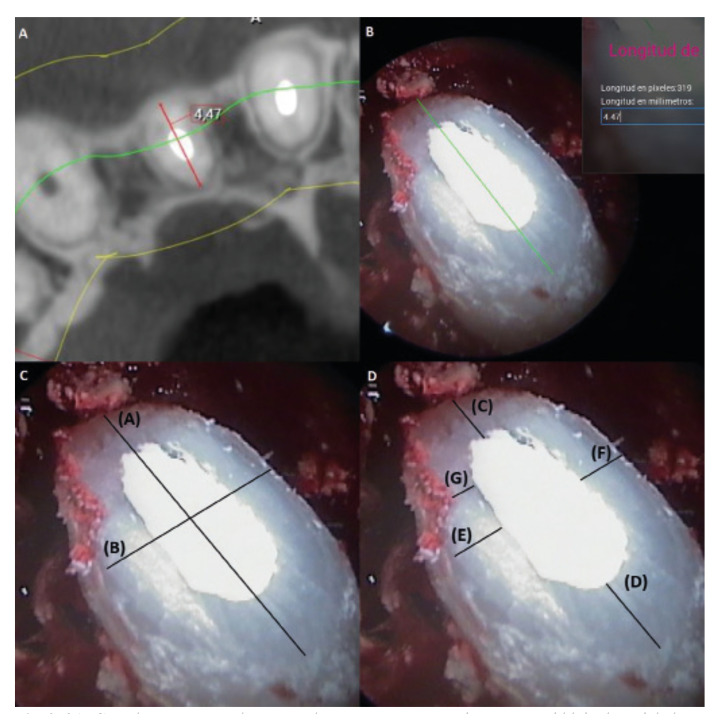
2A: Cone-beam computed tomography measurement: maximum root width in the axial plane. The CBCT image corresponds to Figure 1A; 2B: Calibration of the endoscopic image from the measurement of the maximum root width obtained in the CBCT; 2C: Endoscopic measurements. (A): maximum root width; (B): minimum root width; 2D: Endoscopic measurements. (C, D, E, F): measurements of peripheral dentin thickness; (G): minimum dentin thickness.
